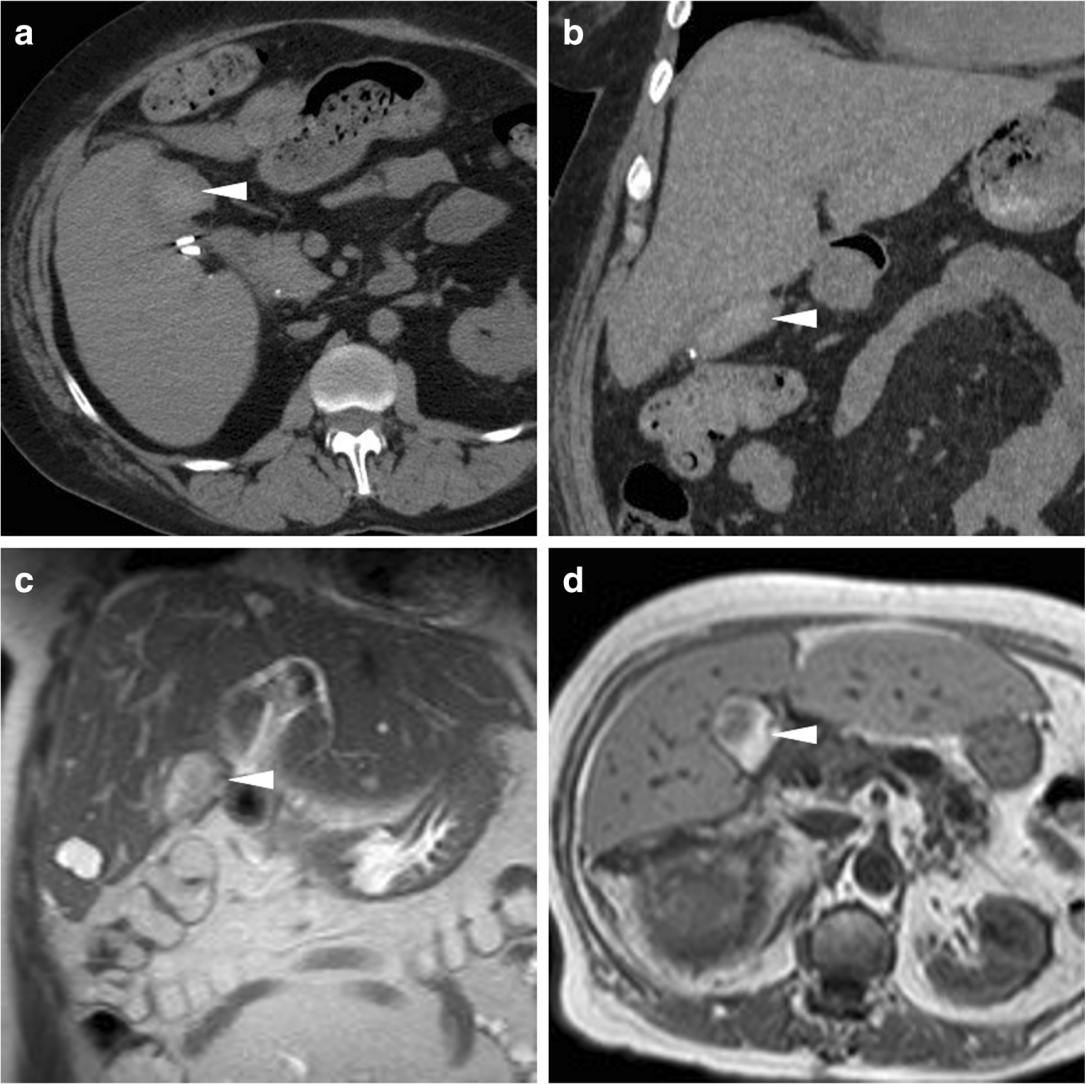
Transient bloody collections in the surgical bed after uncomplicated laparoscopic cholecystectomy. a, b On the 8th postoperative day, unenhanced CT performed showed an ovoid, demarcated collection (*arrowheads*) with high attenuation consistent with resolving blood. c, d In another patient, on the 4th postoperative day, T2- (c) and T1- (d) weighted MR images showed a moderate-sized inhomogeneous collection (*arrowheads*) with signal intensity features consistent with subacute blood. Both findings did not require blood transfusions or any additional treatment
